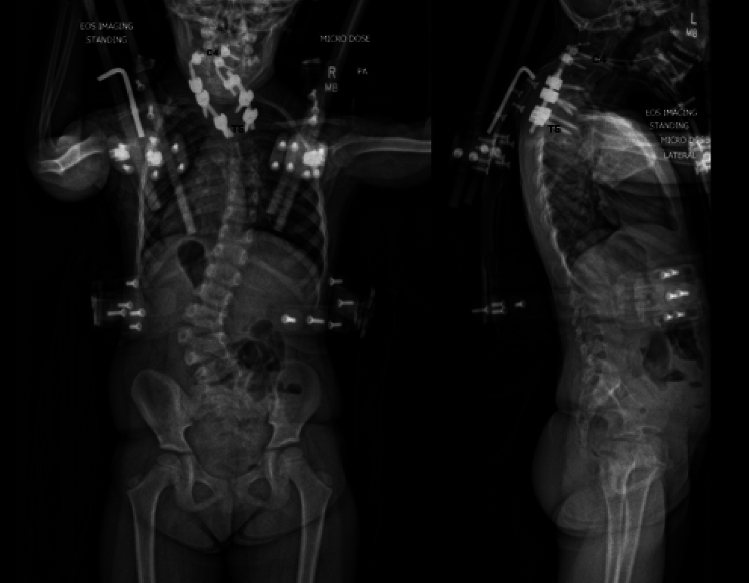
Case 3 Postoperative PA and lateral X-ray of a 4-year-old female with Klippel–Feil syndrome, C1–C2 instability, and congenital scoliosis
